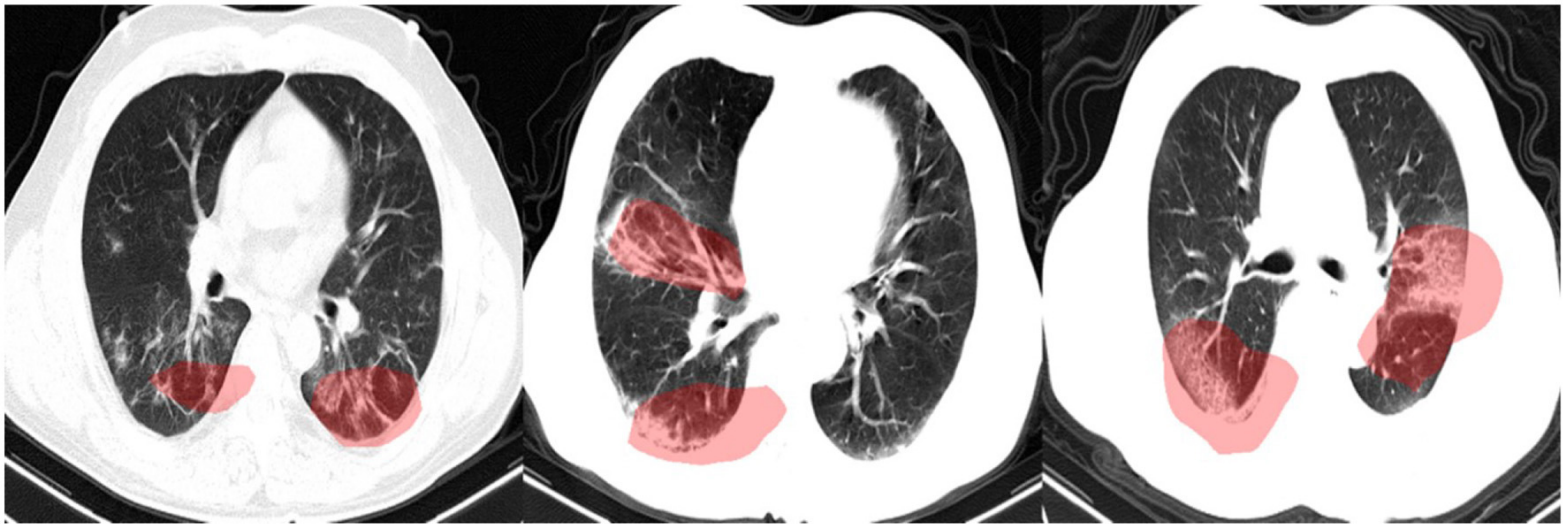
Example chest CT images of COVID-19 cases and their associated critical factors (highlighted in red) as identified by GSInquire (18).
